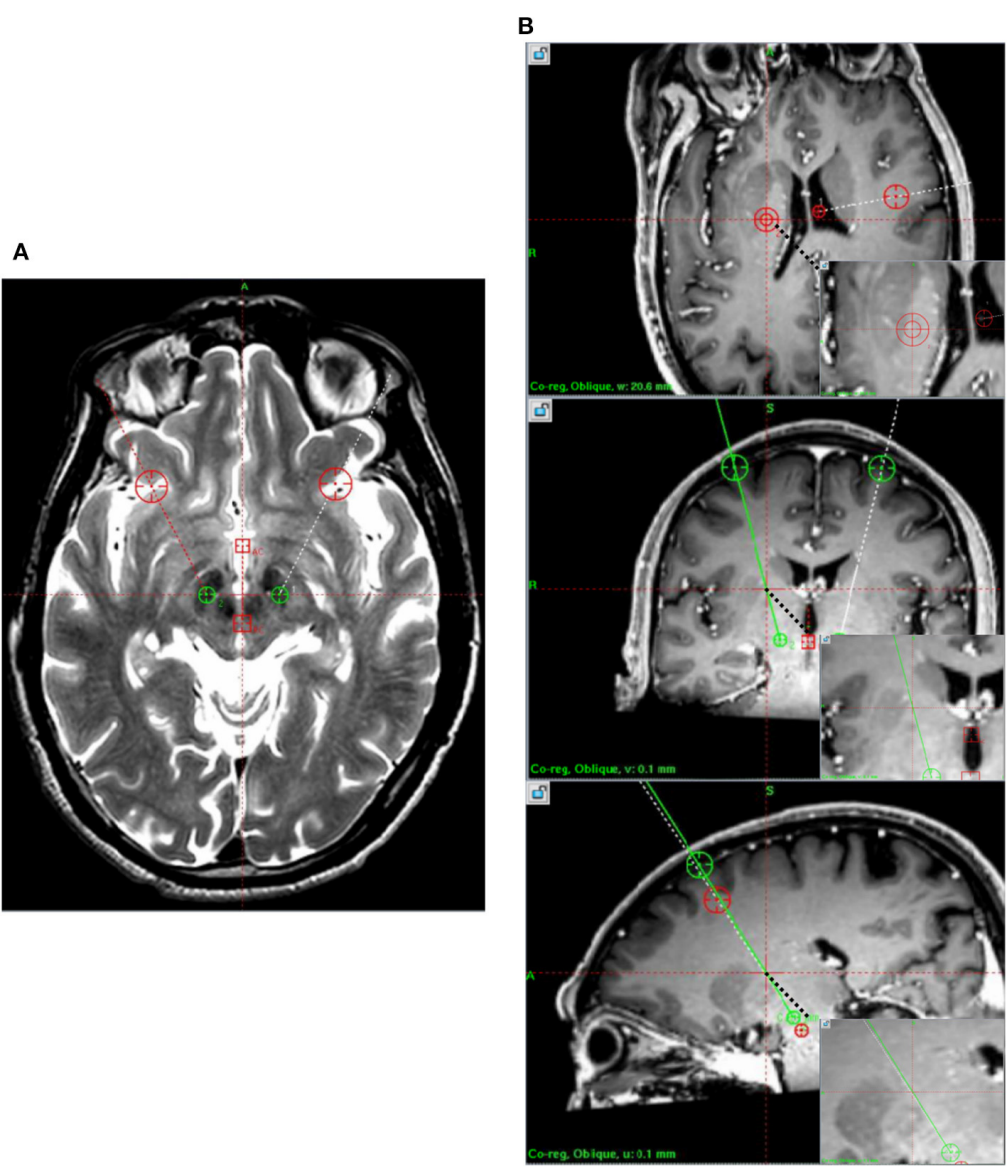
Preoperative magnetic resonance imaging. (A) T2 images demonstrate the target. (B) T1 enhanced the discrimination of the blood vessels.
